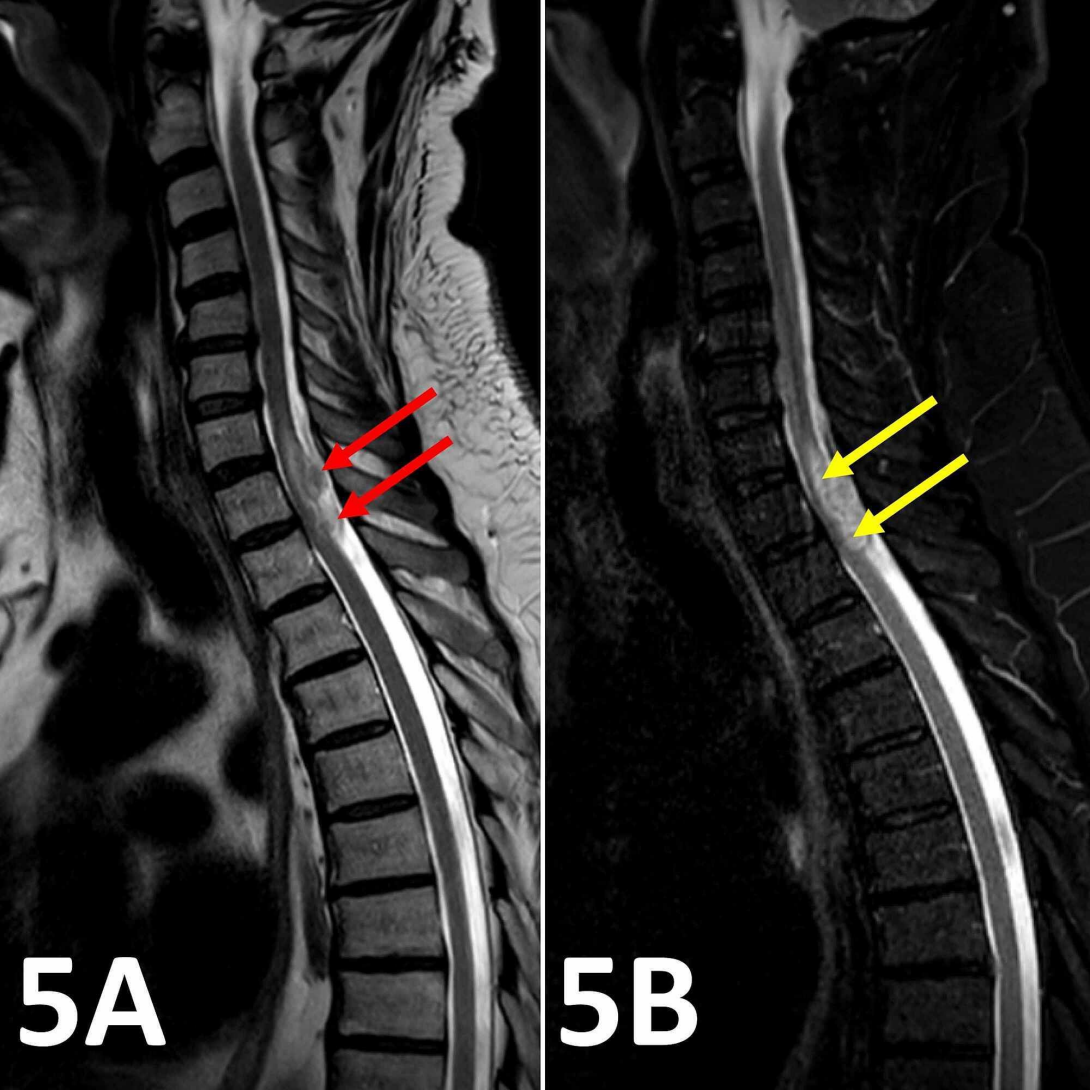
Sagittal T2 and T2 fat-suppressed spinal MRI images. (A) Sagittal T2 spinal MRI image showing the heterogeneously hyperintense characteristics of the two intradural lesions at the T1/T2 vertebral level (red arrows). (B) The T2 fat-suppressed sagittal MRI spine image at the same level reveals no suppression of the high T2 central cord signal signifying mild cord edema (yellow arrows).
